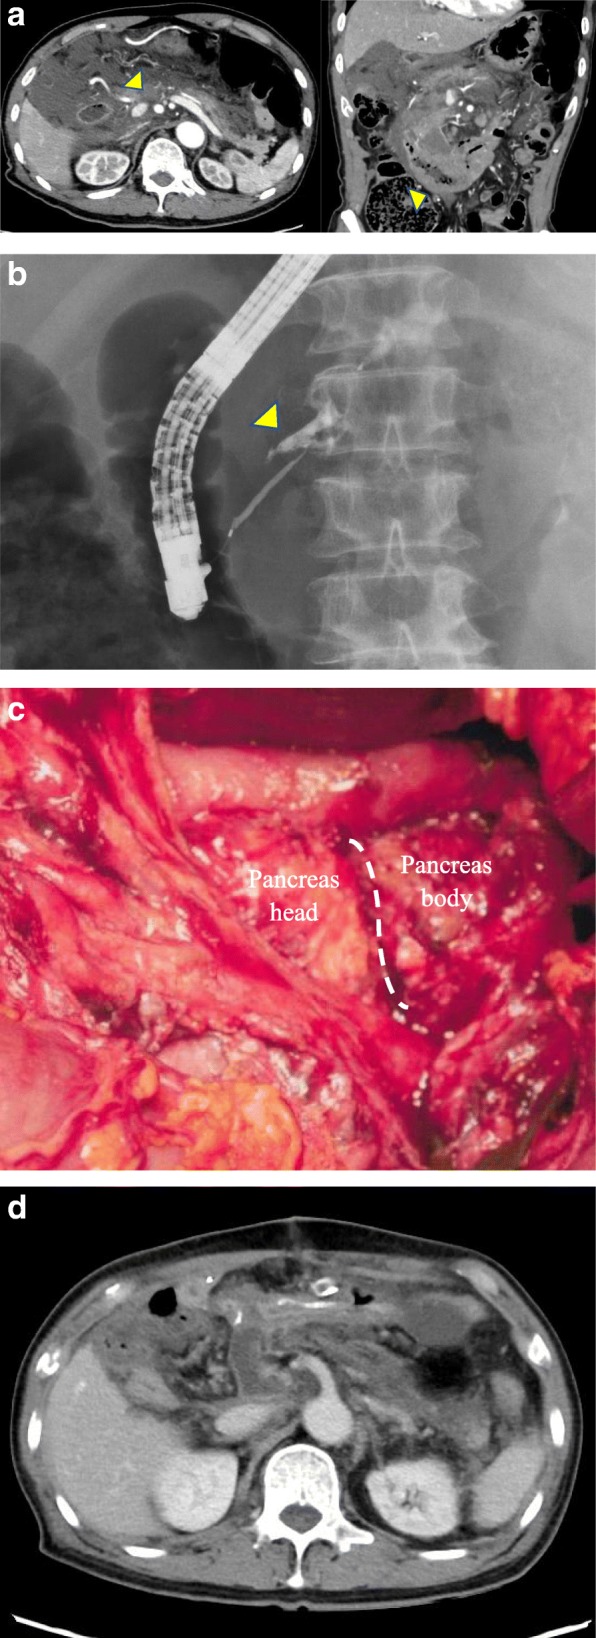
a Abdominal contrast-enhanced computed tomography. Fluid retention around the pancreas (arrow) and wall thickening of the horizontal segment of the duodenum (arrow) are observed. b Pancreatography. Massive leakage of the contrast medium from the main pancreatic duct at the pancreatic head (arrow) is observed. c Pancreas head and body were completely transected. d Abscess formation or pseudoaneurysm formation were not found
